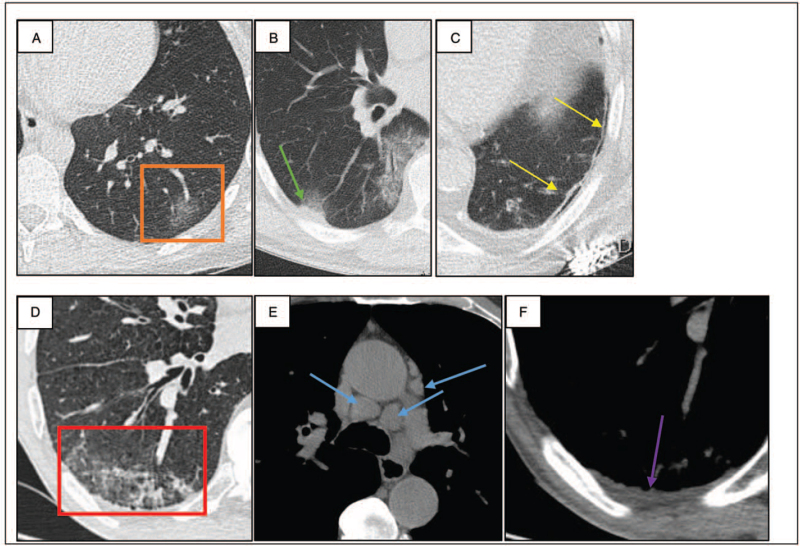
Top 3 typical and atypical CT findings. A, Nodular ground-glass opacity: appearance of a hazy nodular ground-glass opacity (orange box), typically bilateral, and in the peripheral and lower parts of the lungs in COVID-19 pneumonia. B, Pleural thickening: adjacent to the peripheral opacities there is frequently a subtle focal pleural thickening confirming the “organizing pneumonia-like” aspect. C, Subpleural bands: axial CT image in a COVID-19 positive patient shows subpleural bands (yellow arrows) basal in the lung. Subpleural bands are defined as thin curvilinear opacities (1–3 mm thickness) lying subpleural and parallel to the pleural surface. Centrilobular nodules (D), enlarged lymph nodes (E), and pleural effusion (F) are the top 3 atypical CT findings: superimposed aspiration pneumonia in a COVID-19-positive patient with known CPFE (centrilobular emphysema and combined systemic sclerosis/scleroderma-related fNSIP). Axial CT image shows peripheral areas of ground-glass opacity and some clustered centrilobular nodules (red box) basal in the right lung. The mediastinal window shows enlarged mediastinal lymph nodes (blue arrows) and a small pleural effusion (purple arrow) basal in the right lung. The combined findings of nodules, enlarged lymph nodes, and pleural effusion are suggestive for bacterial superinfection. COVID-19 = coronavirus disease 2019, CT = computed tomography.
